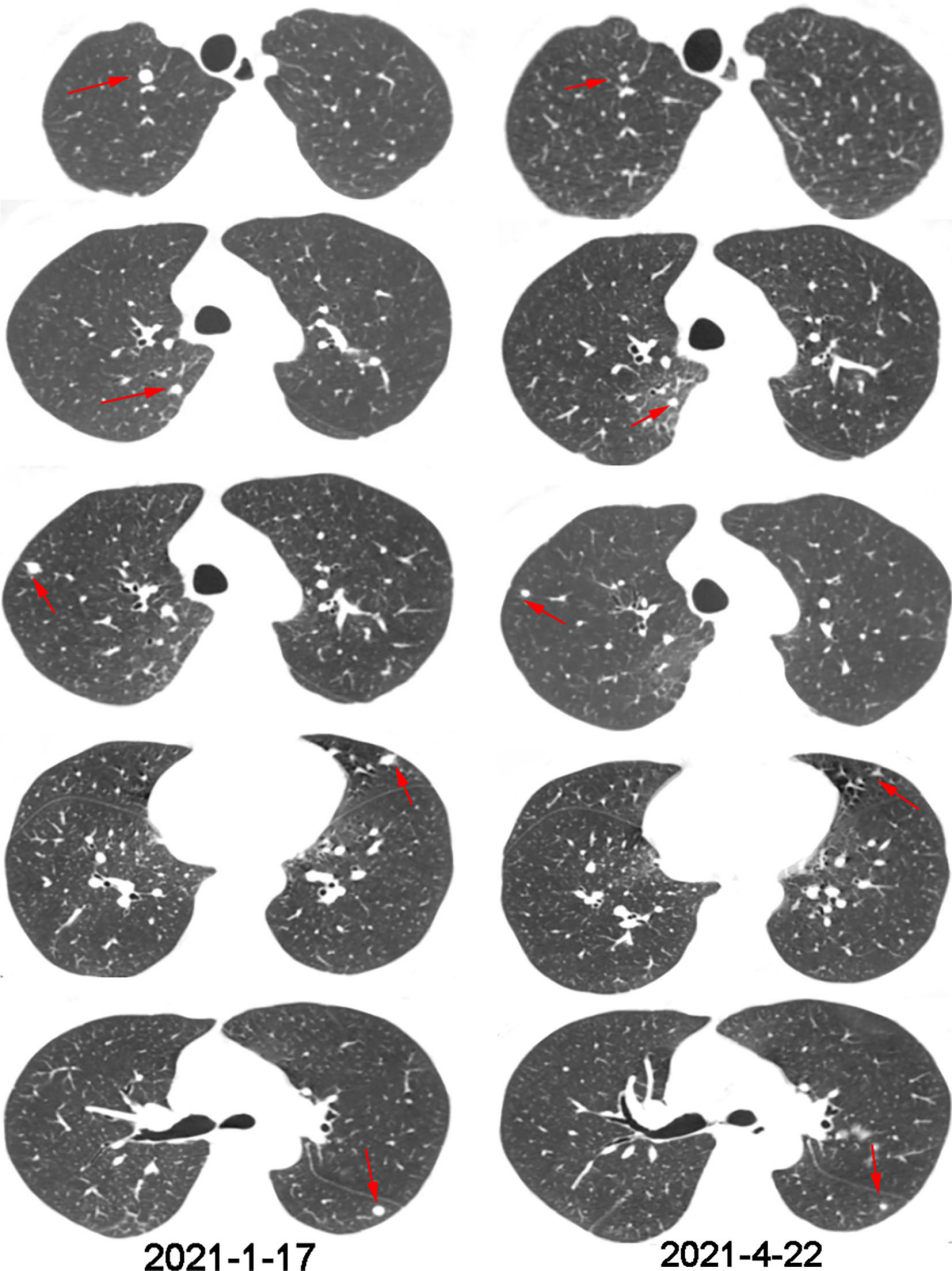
Chest CT scans before and after one cycle of triple-combination therapy and two cycles of sintilimab treatment.
